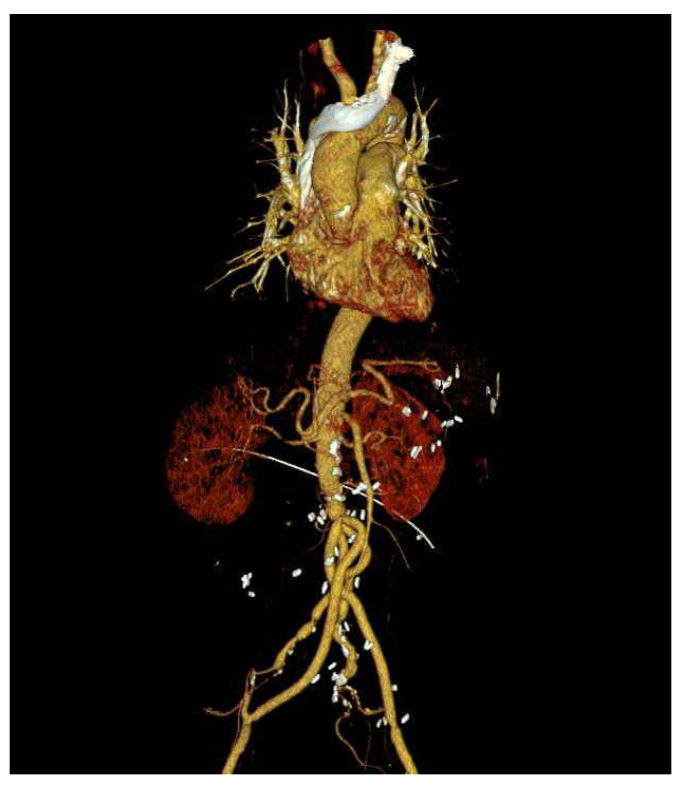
Postoperative CT scan of abdominal aortic aneurysm open repair.
